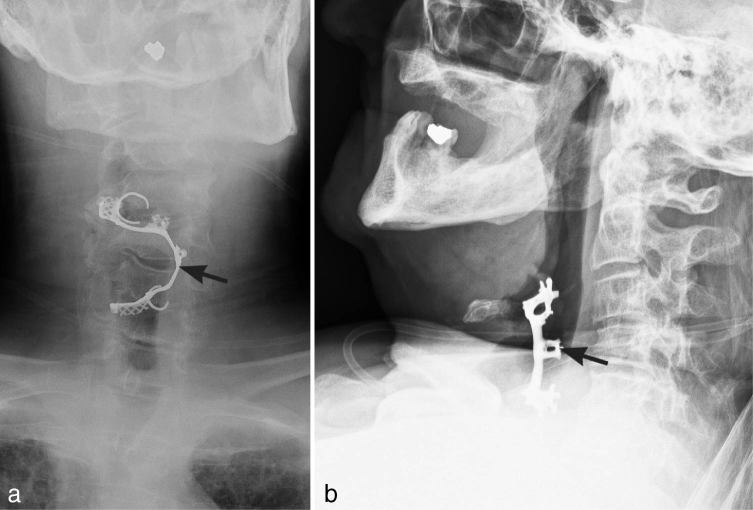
Anteroposterior (a) and lateral (b) neck soft tissue radiographs show a denture (arrows) in the hypopharynx. Mild distension of the pharynx with air, more apparent on the lateral view (b), is likely secondary to mass effect of the foreign body on the larynx.
